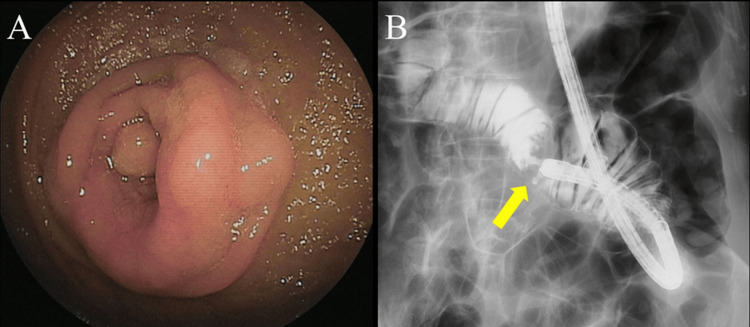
Small bowel endoscopy and radiograph A: Small bowel endoscopy revealed a localized-ulcerating tumor encircling the jejunum; B: Small bowel radiograph showing an apple core sign in the jejunum, 40 cm from the ligament of Treitz (arrow)
